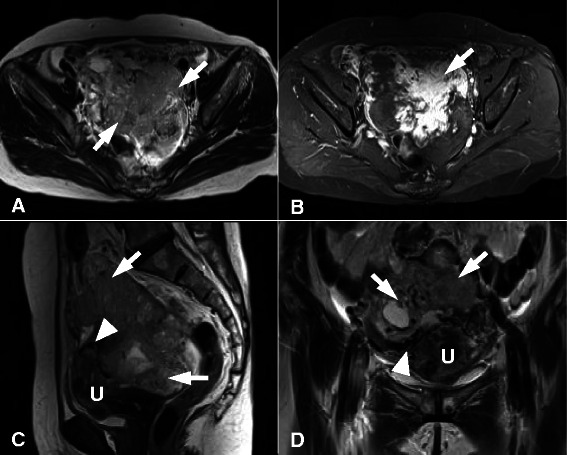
Pelvic magnetic resonance image of the pedunculated subserosal leiomyosarcoma of the uterus. a Axial T2-weighted image showing a large pelvic mass (arrows) with heterogeneous signal intensity. b Contrast-enhanced fat-suppressed T1-weighted image showing marked heterogeneous enhancement (arrow) of the mass. c, d On sagittal (c) and coronal (d) T2-weighted images, the mass (arrows) abutted the uterus (U). Note the bridging vessels (arrowheads) between the uterus and the juxta-uterine mass
